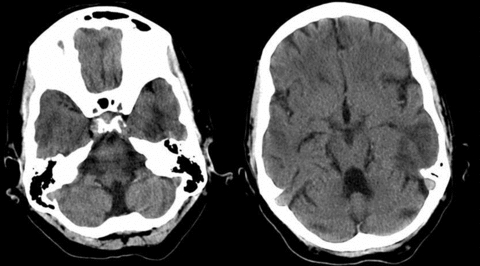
CT head upon presentation. Noncontrast CT of the head showing bilateral temporal hypodensities, left greater than right.
